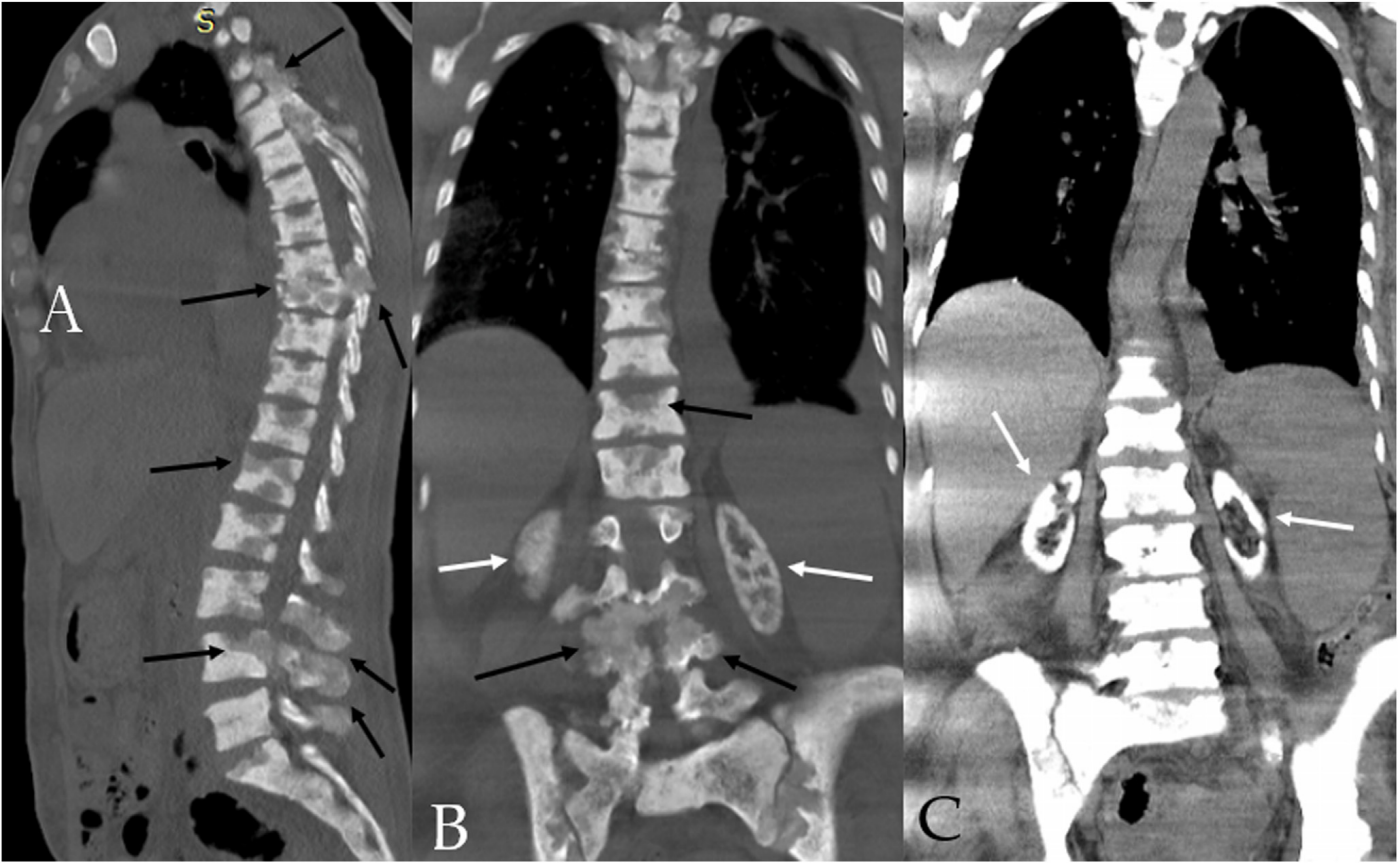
CT scan; bone (A+B) and abdominal (C) windows shows the following:1)Diffused lytic lesions along all vertebrae with vertebral erosions and destruction of vertebral bodies and spinous processes (black arrows).2)Cortical kidney calcifications (white arrows)3)Hepatosplenomegaly.
